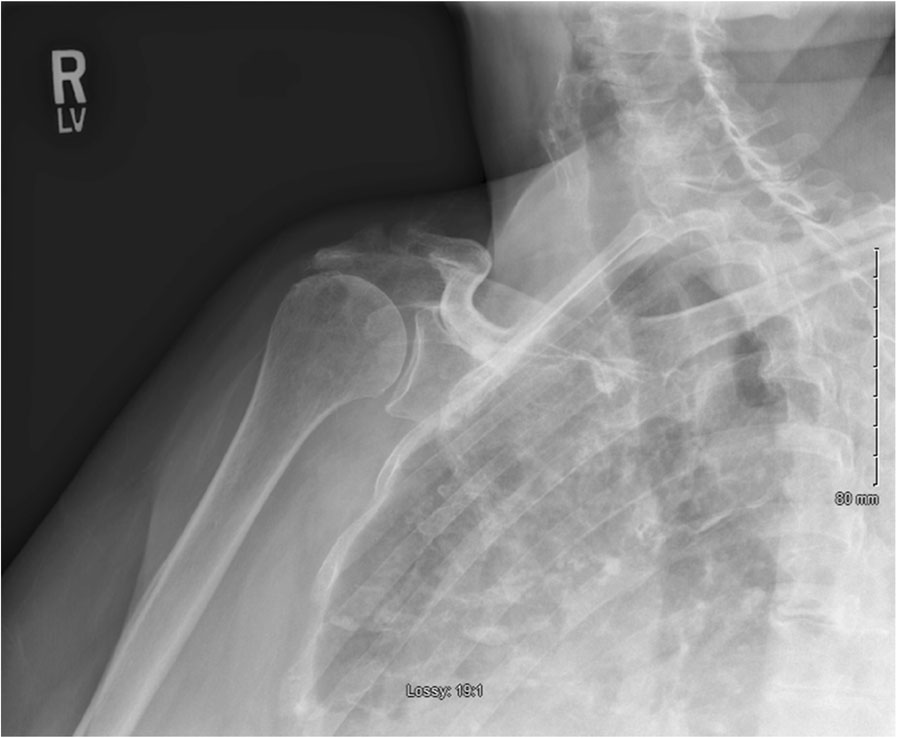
AP pre-operative radiograph of the right shoulder demonstrating superior migration of the humeral head and early acetabularization of the acromion
